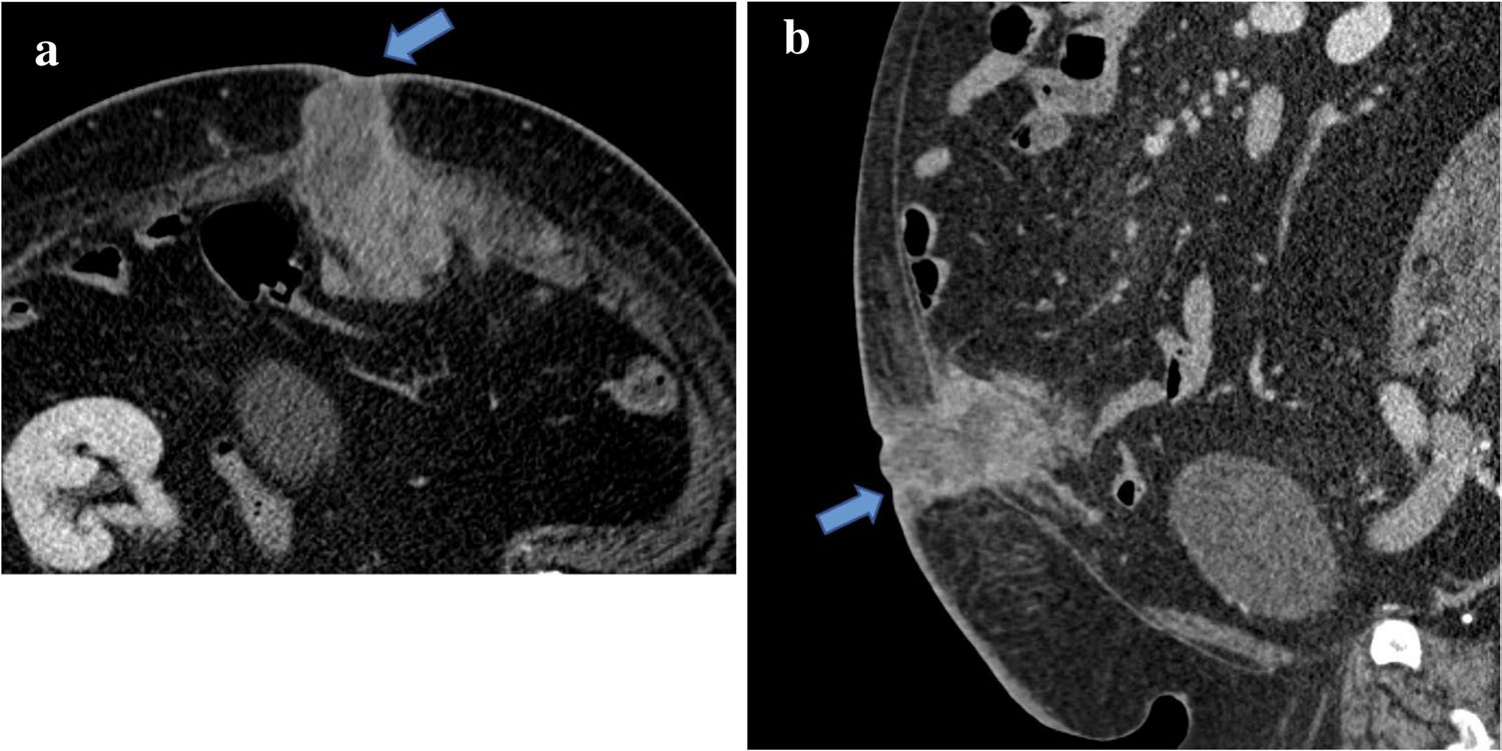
Seventy-one-year-old male with history of hepatocellular carcinoma and biopsy-proven umbilical abdominal wall metastasis. Postcontrast axial (a) and sagittal (b) CT of the abdomen demonstrates a heterogeneously enhancing mass at the anterior abdomen, with portions that involve the periumbilical abdominal wall and portions that are intraperitoneal and inseparable from small bowel
